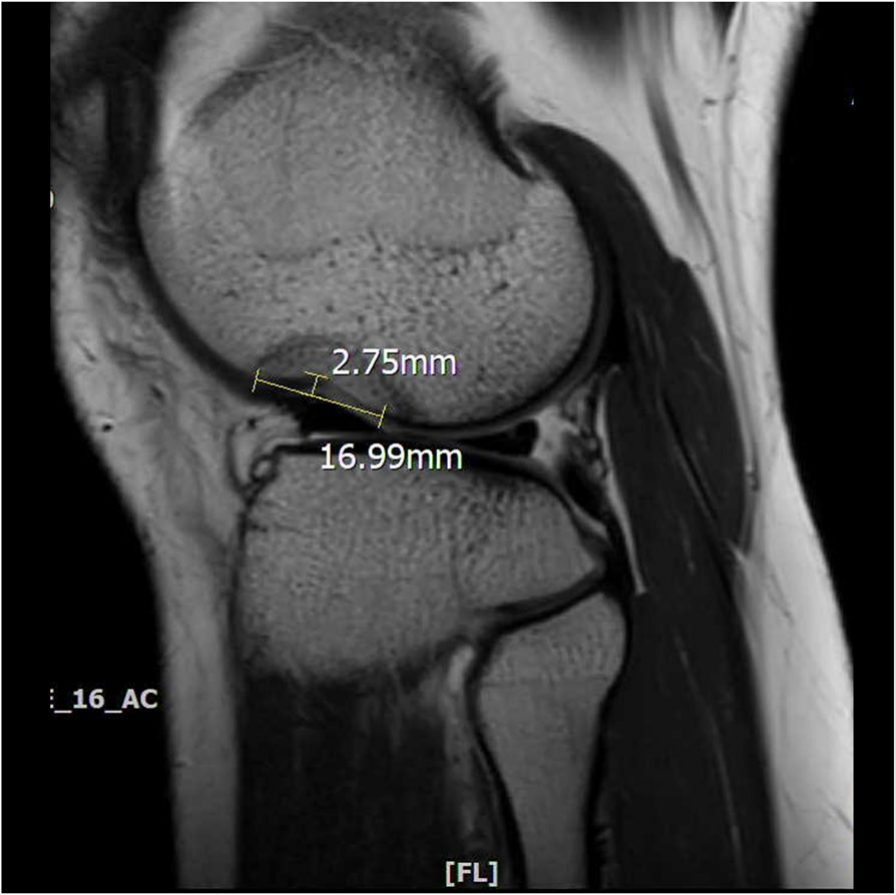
Measurement of the lateral femoral notch sign. The notch sign was measured on a sagittal T1-weighted magnetic resonance imaging based on the tangent method following a previous study [3]
